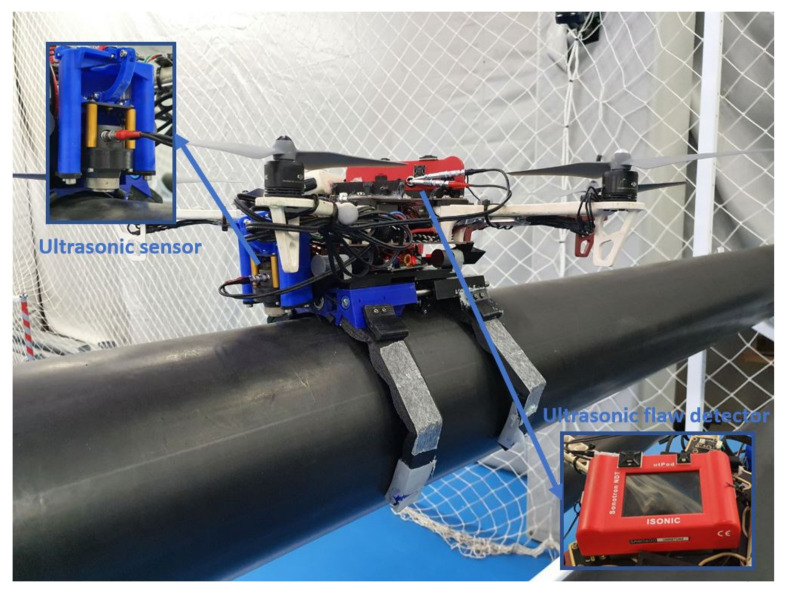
The image shows the complete system with an ultrasonic sensor and the flaw detection computer.
